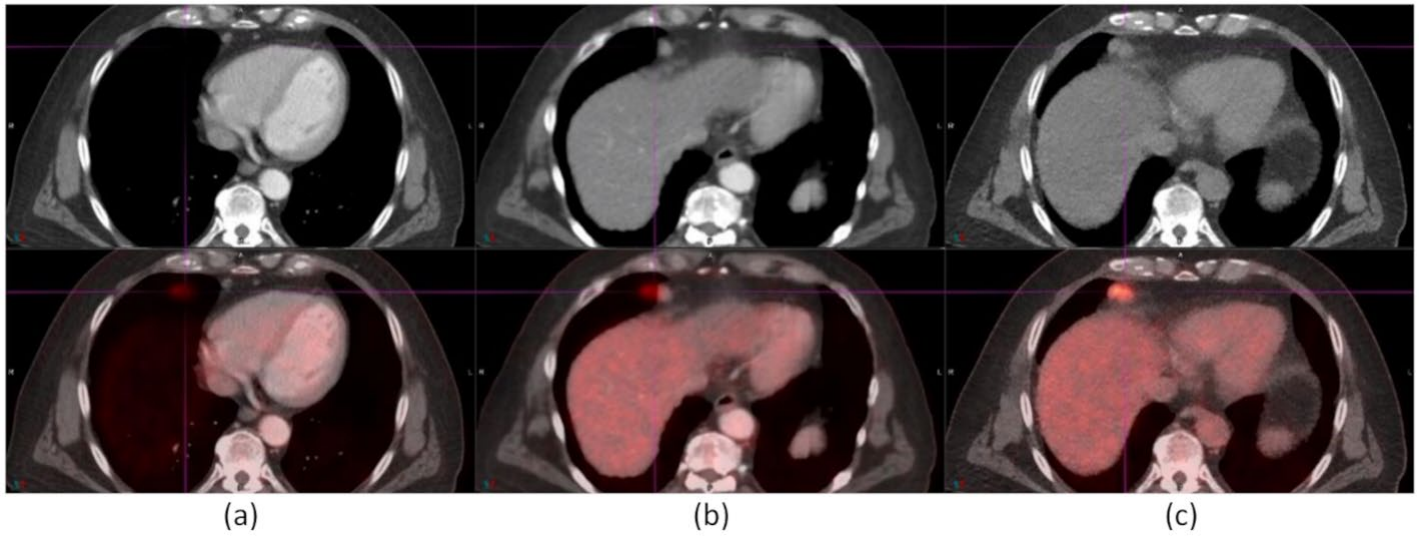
Two anterior diaphragmatic lymph nodes, measuring 1.0 cm and 1.2 cm, were identified on DDG CT (c) but were indistinguishable in both baseline (a) and AIR (b). The SUV_max_ values for these nodes were 3.73 (baseline) and 5.26 (AIR), to 7.18 and 6.37 on DDG CT. Notably, the shape of the vertebral body and the locations of the ribs in AIR (b) differed from those observed in baseline (a) or DDG CT (c)
